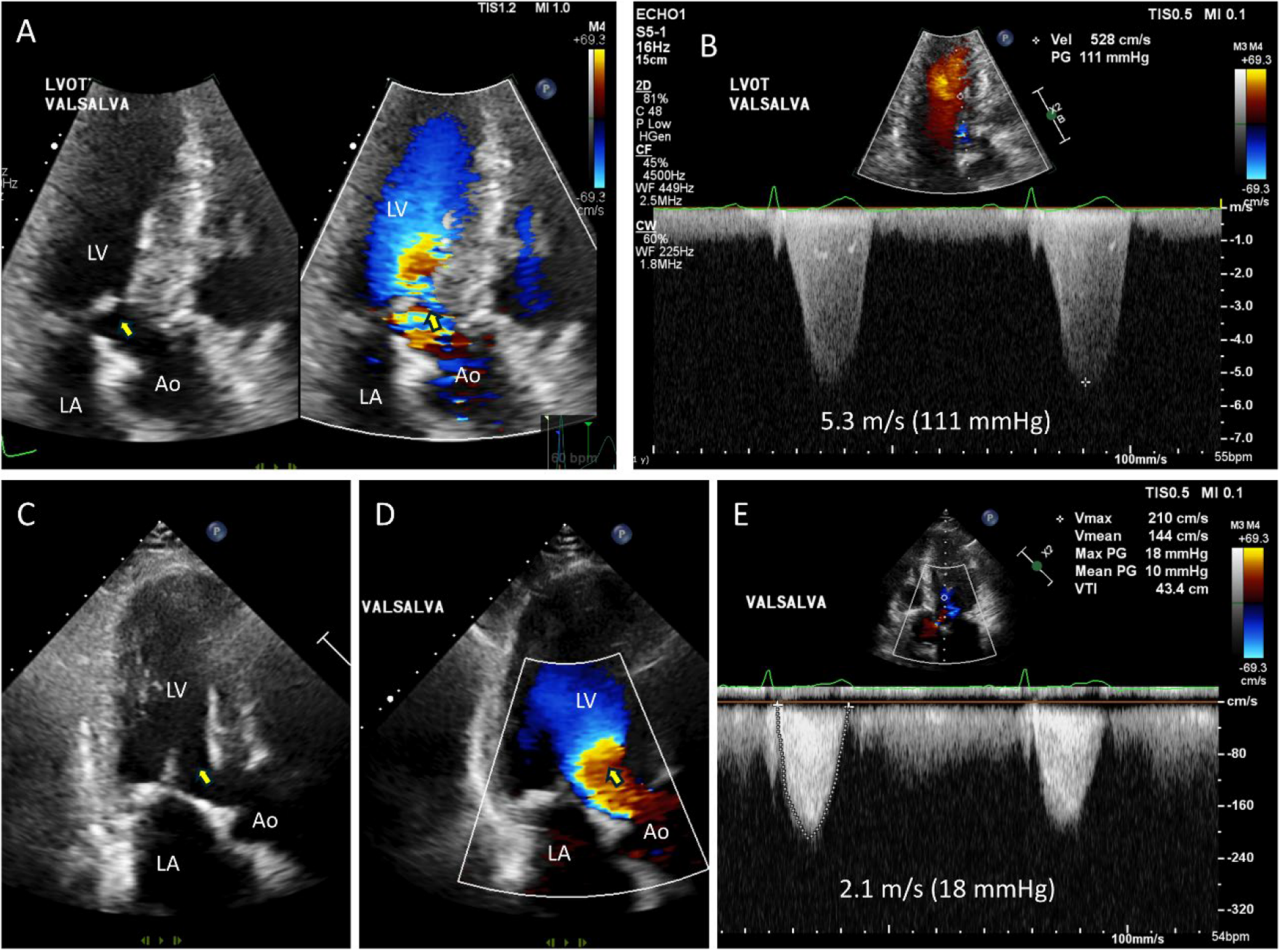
A representative case showing the effect of a cardiac myosin inhibitor in a sigmoid septum with significant left ventricular outflow tract (LVOT) obstruction. An 82-year-old man experienced intractable exertional dyspnea despite multiple medications including β-receptor blocker. Echocardiography showed (A) sigmoid septum and prominent SAM-septal contact resulting in (B) very high peak velocity at the LVOT. (C, D) Loss of SAM-septal contact occurred with additional treatment with a cardiac myosin inhibitor, resulting in (E) effective relief of LVOT obstruction
